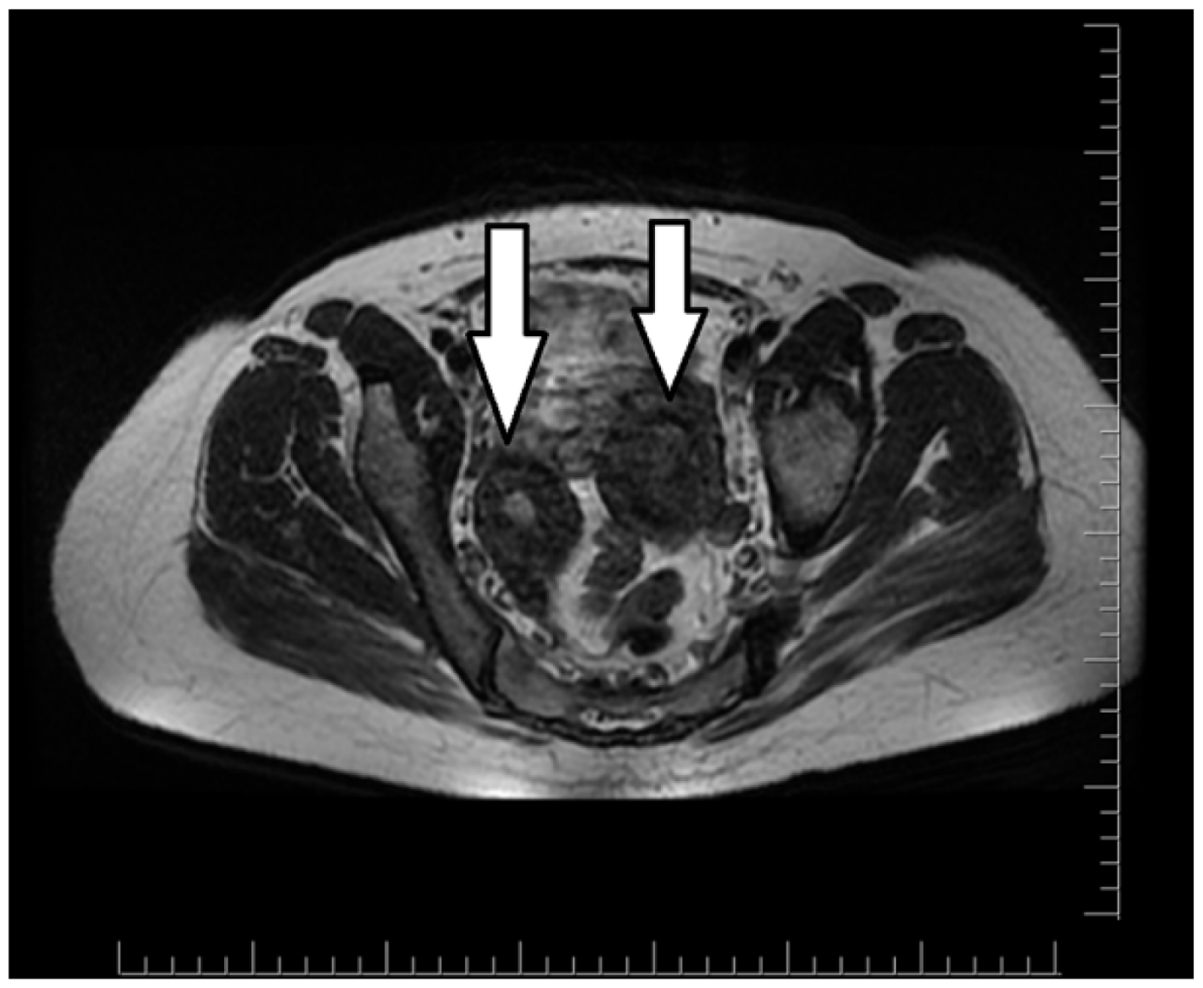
MRI: Axial T2-weighted image shows the presence of a normal didelphic uterus at the 6-month follow-up (white arrows).
